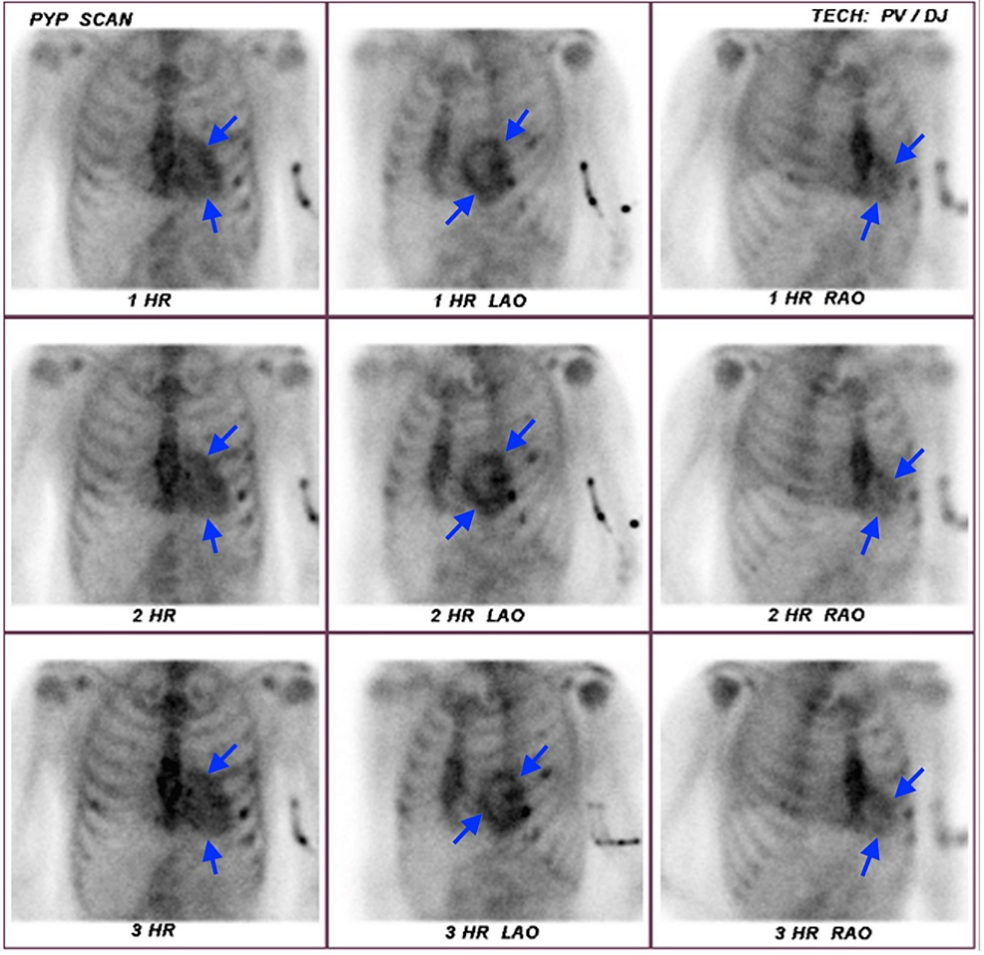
99mTc-PYP scintigraphy showing cardiac radiotracer uptake that is more pronounced than locoregional osseous uptake. 99mTc-PYP: technetium-99m pyrophosphate.
